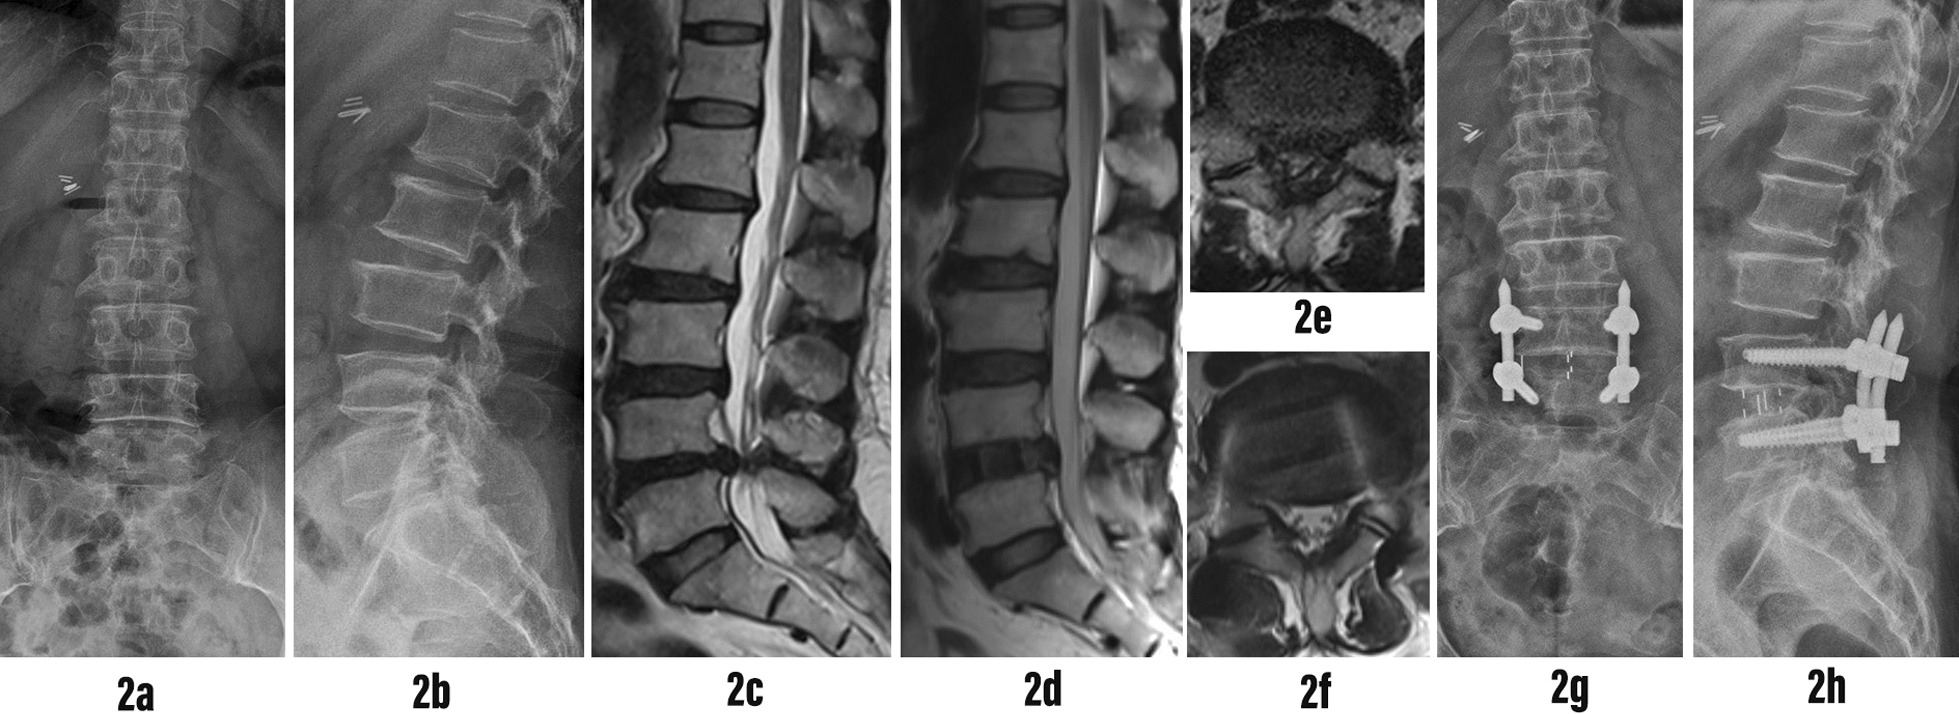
Typical case 2: A 76-year-old female presented with low back discomfort and right lower limb numbness for more than 2 years, which aggravated for 1 week. Diagnosis of L4-5 spinal stenosis. L4-5 single segment OLIF combined with posterior L4-5 percutaneous internal fixation was performed. 2a, 2b Anteroposterior and lateral X-ray films of lumbar spine before operation showed lumbar degeneration. 2c Preoperative sagittal T2 MRI of the lumbar spine showed severe spinal stenosis at L4-5 segment. RNRs was seen above the stenosis plane. 2d Postoperative sagittal T2 MRI of the lumbar spine showed that the RNRs above the L4-5 surgical segment disappeared. 2e, 2f Axial T2 MRI of lumbar spine before and after operation showed that the dural sac CSA increased significantly after OLIF indirect decompression. 2g, 2h At the last follow-up, the X-ray film of the lumbar spine showed that the L4-5 pedicle screw was fixed, the cage was placed in the middle and the position was good, and the height of the L4-5 intervertebral space was recovered well
